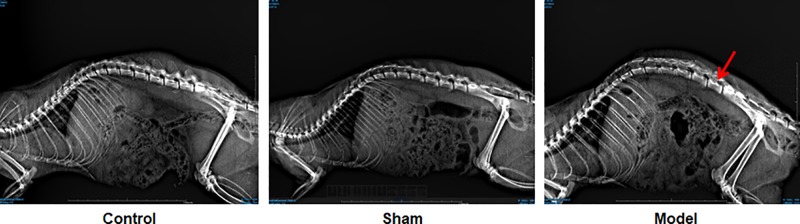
X-rays of vertebrae 3 months after modeling operation Arrow indicated narrowed intervertebral space.
